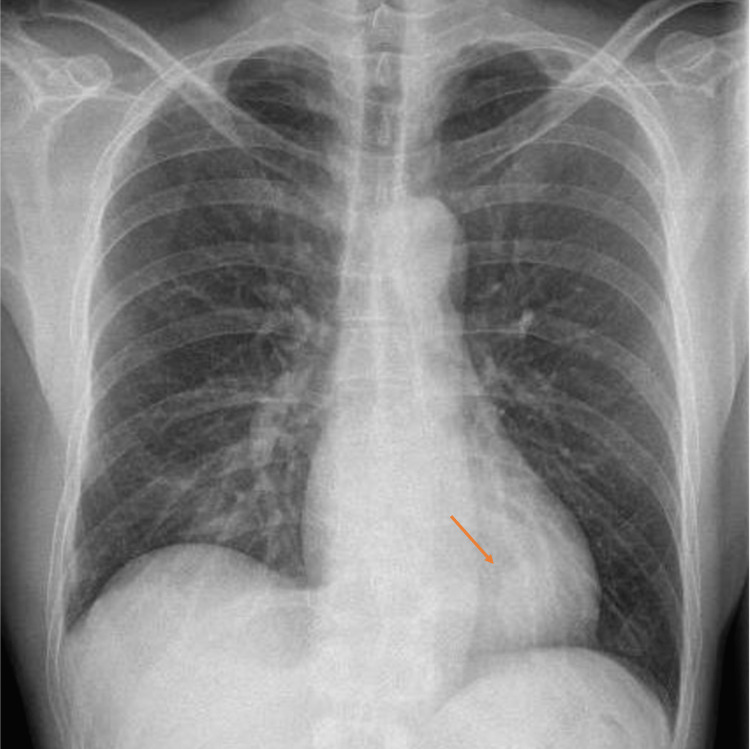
Chest radiograph on admission.
